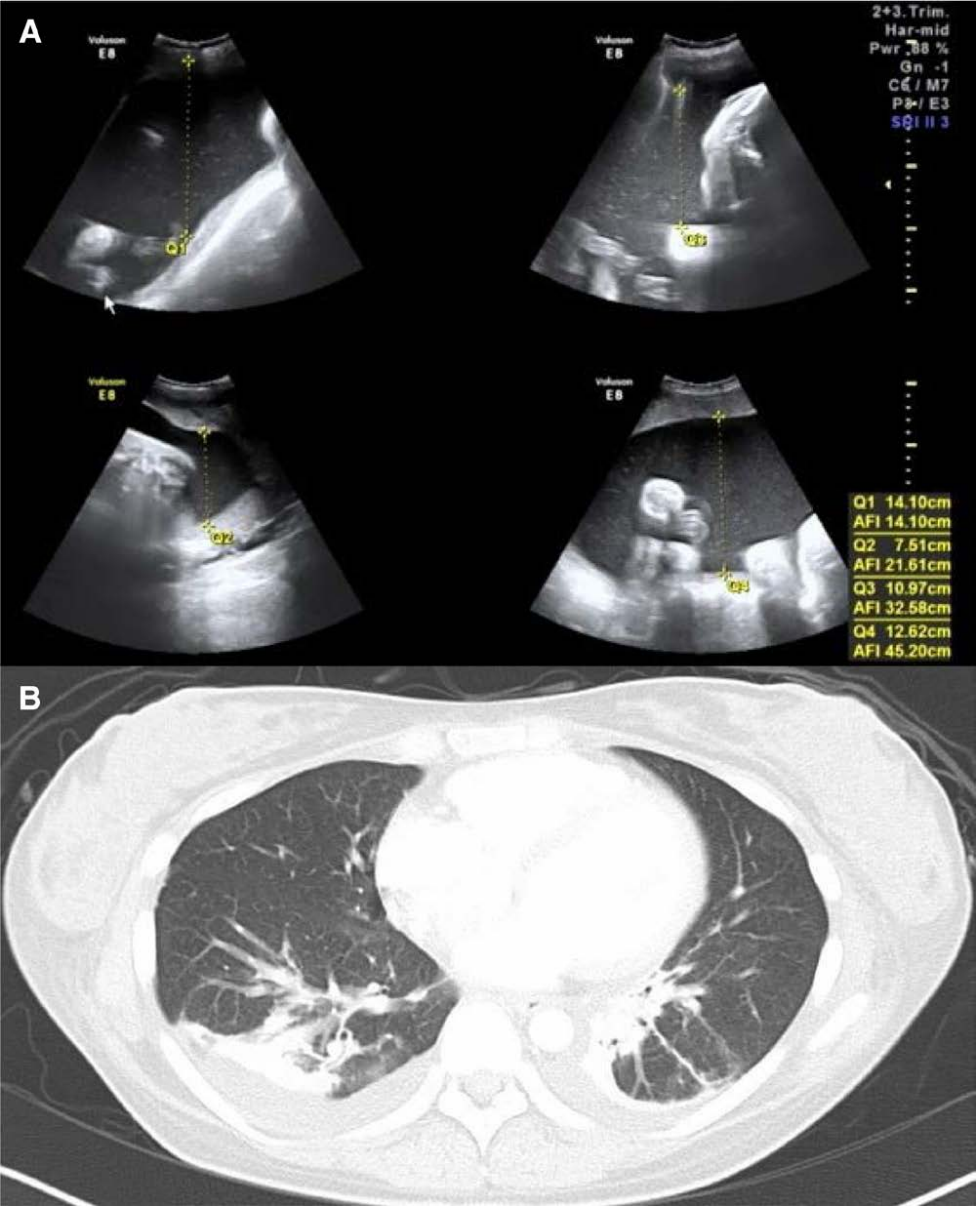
(A) Fetal ultrasound image at 36 weeks of gestation showing polyhydramnios. (B) Chest CT at 2 days after the delivery showed bilateral pleural effusions and mild pulmonary edema. CT = computed tomography.
